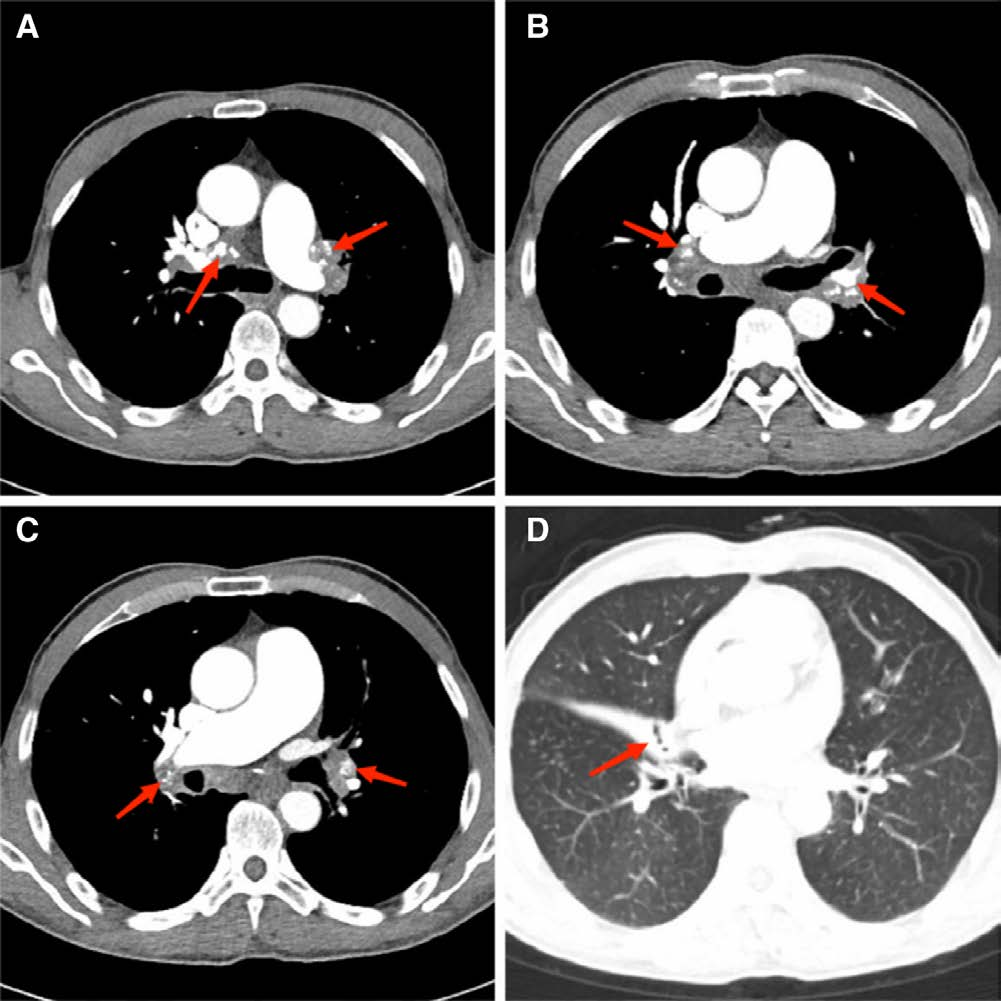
Contrast-enhanced chest CT findings upon admission. (A–C) Bilateral hilar and mediastinal lymph nodes show heterogeneous enlargement with poorly defined margins, and partial calcification is observed (red arrows). (D) Atelectasis of the right middle lung lobe is evident (red arrow). CT = computed tomography.
